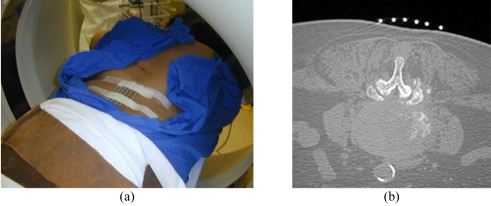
Grid method of surface marking for a lumbar biopsy. (a) Photograph shows placement of a grid of radio-opaque skin markers to help determine the needle entry point. (b) Preliminary axial CT image taken with the patient prone shows the grid of skin markers at the selected level. The most appropriate entry point can then be selected and surface-marked.
